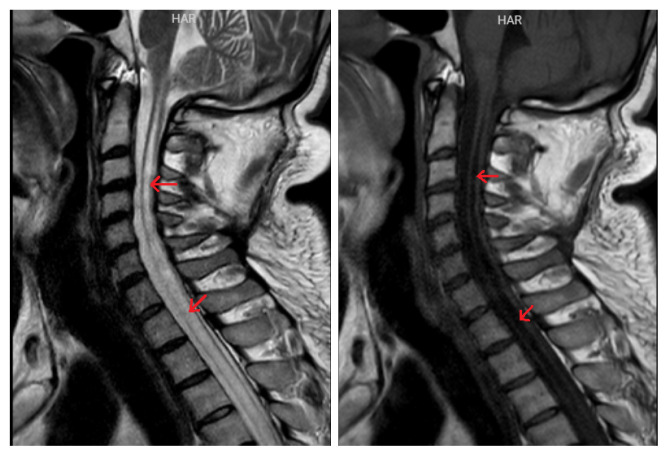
Magnetic resonance imaging of the patient with syringomyelia in the cervical region. Red arrows: Cervical spinal cord syrinx.
